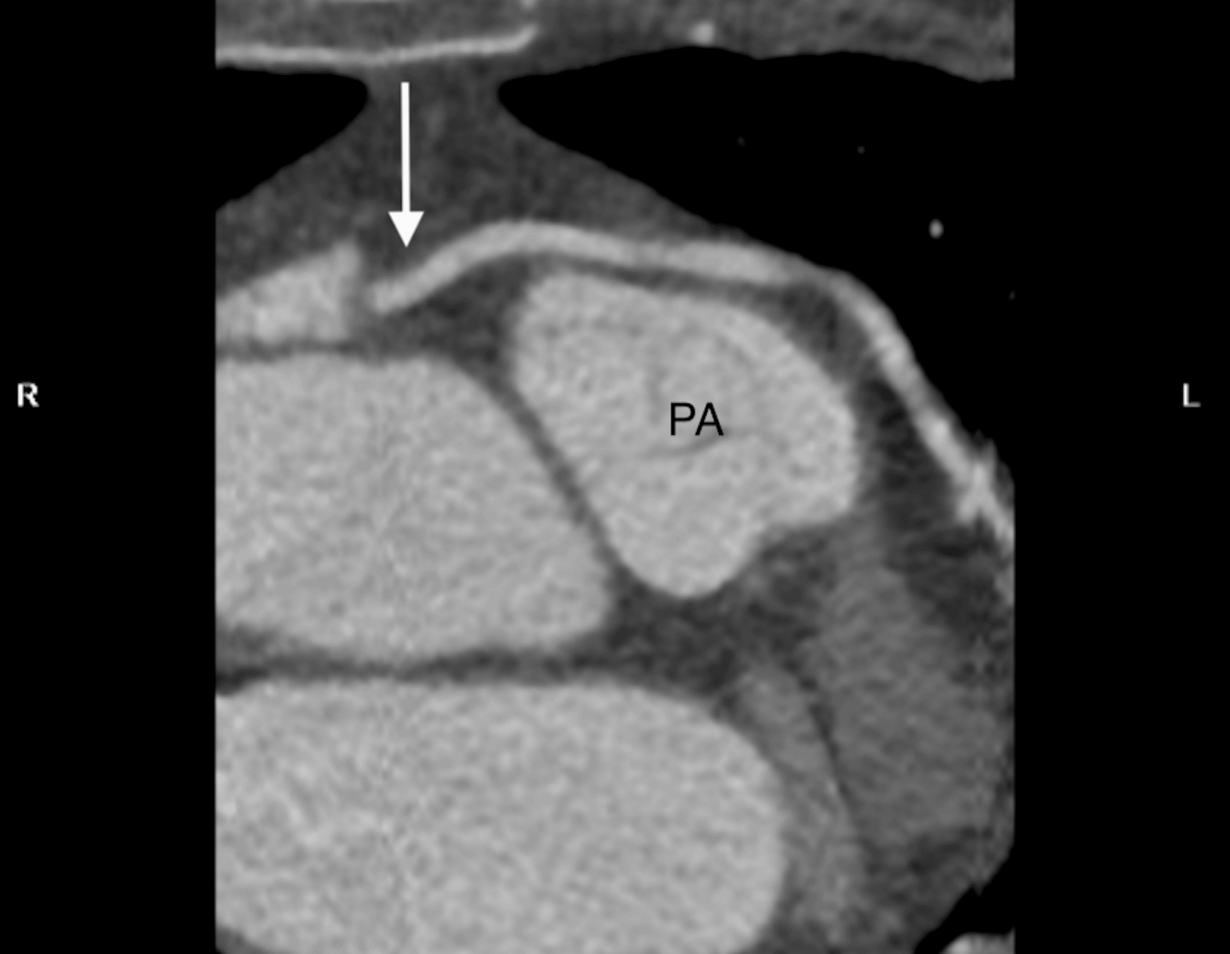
2D coronary CTA Two-dimensional coronary computed tomography angiography (CTA) showing the anomalous large left main coronary artery (LMCA) (white arrow) arising from the right sinus of Valsalva (RSV) and coursing superiorly and anteriorly to the pulmonary artery (PA). The image highlights the vessel’s low-risk anterior course and relationship to the great vessels, supporting conservative management.
